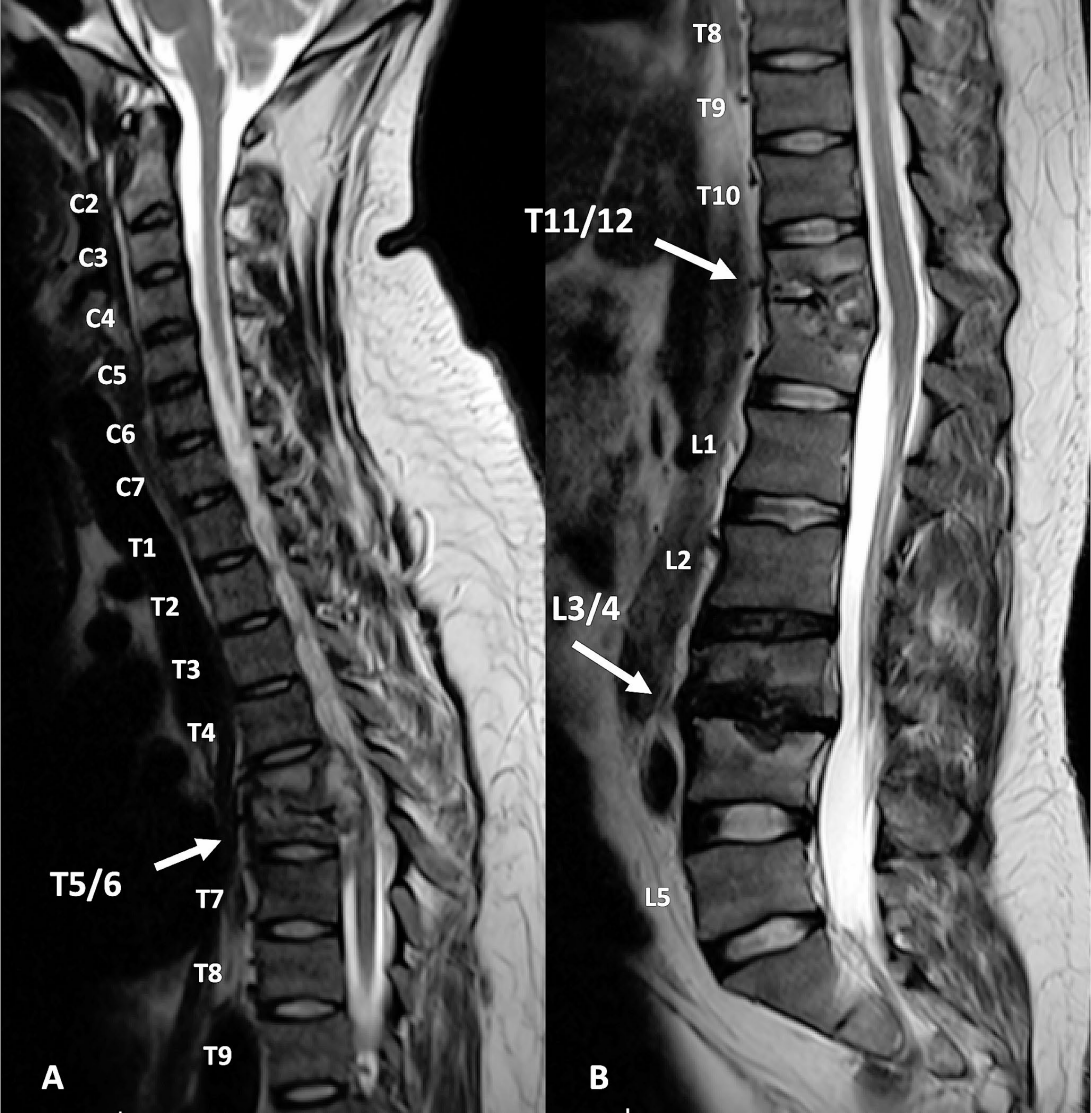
A: T2-weighted sagittal MRI of the upper vertebral column showing T5/6 endplate destruction with mild kyphotic deformity. Intervertebral disc space integrity lost, but low signal disc still visualized. Heterogenous T2 signal soft tissue effacing the anterior CSF space and impressing the anterior cord. B: T2-weighted sagittal MRI of the lower vertebral column showing T11/12 endplate destruction with moderate kyphotic deformity. Intervertebral disc space integrity lost, but low signal disc still relatively maintained. Heterogenous T2 signal soft tissue minimally effacing the anterior CSF space and without cord compromise. L3/4: Endplate destruction with moderate kyphotic deformity. Intervertebral disc space integrity lost, but low signal disc still relatively maintained. Heterogenous T2 signal soft tissue minimally effacing the anterior CSF space without cauda equina compromise
